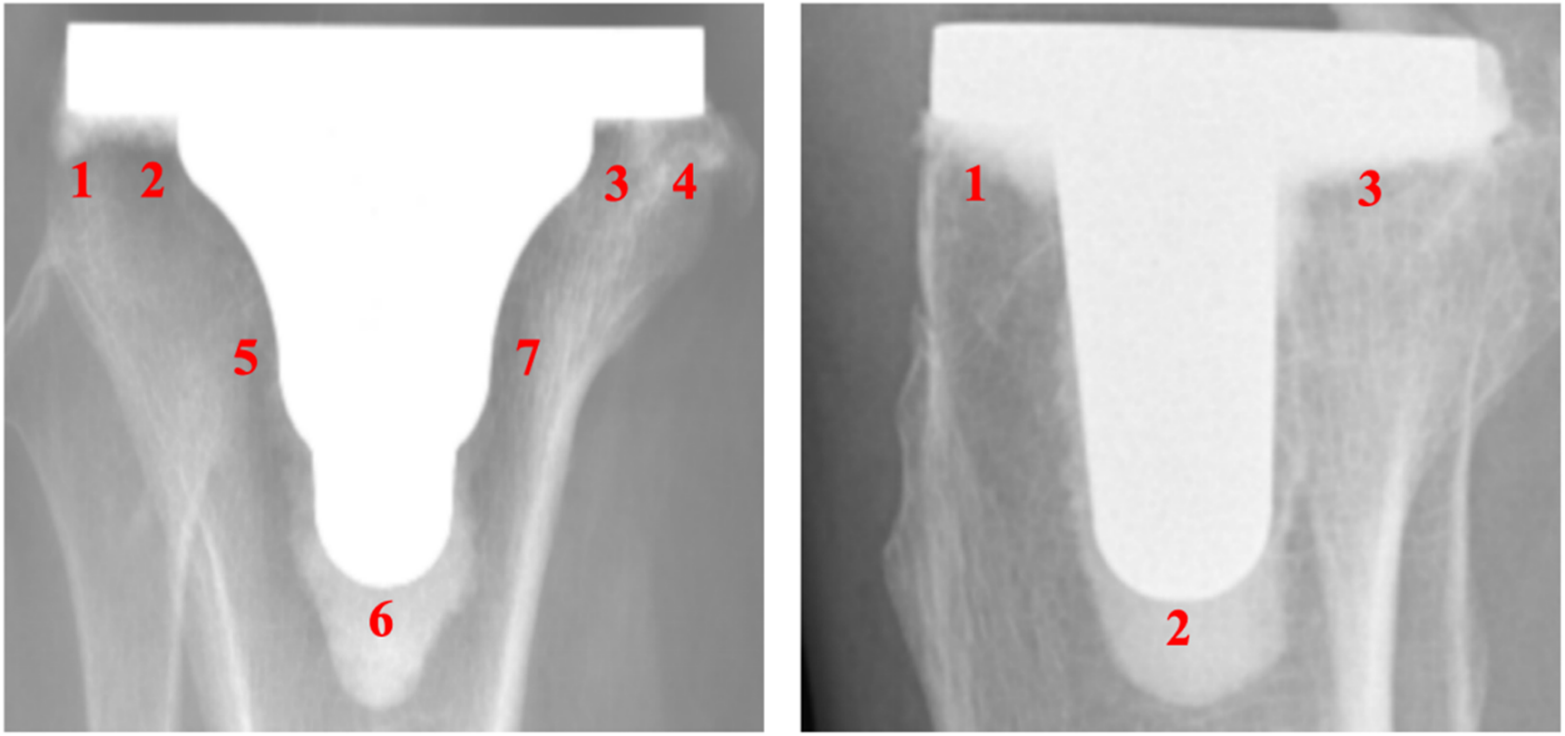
Knee joint AP and lateral x-ray images, divided according to the American knee association TKA postoperative x-ray evaluation system (KSRES score).
